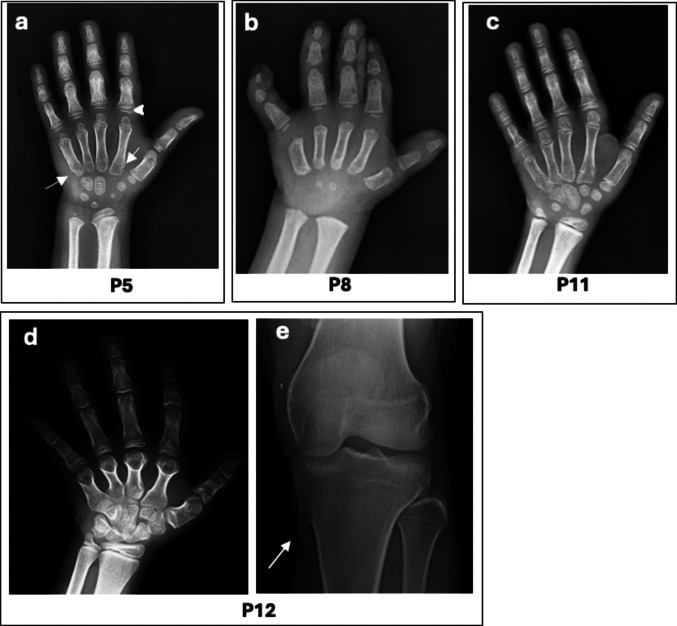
The radiograph of P5 with acromicric dysplasia at 5.4 years showed cone-shaped epiphyses (arrowhead), notching of the second and fifth metacarpals (arrows), short and stubby proximal and middle phalanges, and delayed bone age (a). Radiographs of P8 with Weill–Marchesani syndrome (WMS) type 1 at 3 years of age (b) and P11 with WMS type 4 at 10.8 years of age (c) showed short tubular bones and marked shortening of the fifth middle phalanges. P8 also had delayed bone age, broad and short proximal phalanges, and a broad fifth metacarpal bone (b). The hand radiograph of P12 with Albright hereditary osteodystrophy at 8.6 years of age showed short and broad metacarpal bones (d), and heterotopic ossification in the medial region of the left tibia (arrow) was observed at 15.2 years of age (e)
